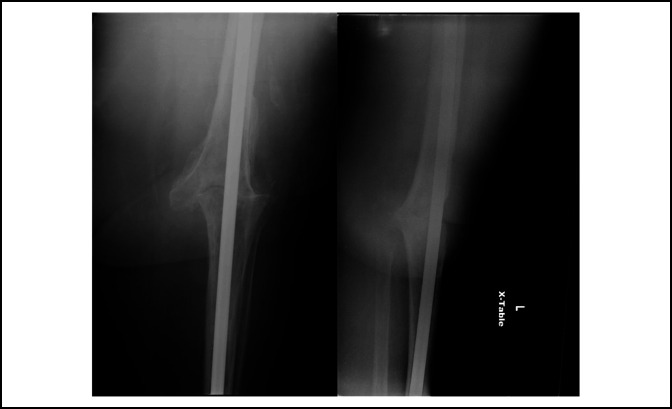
Anteroposterior and lateral radiographs of a knee following arthrodesis with a long intramedullary nail.
